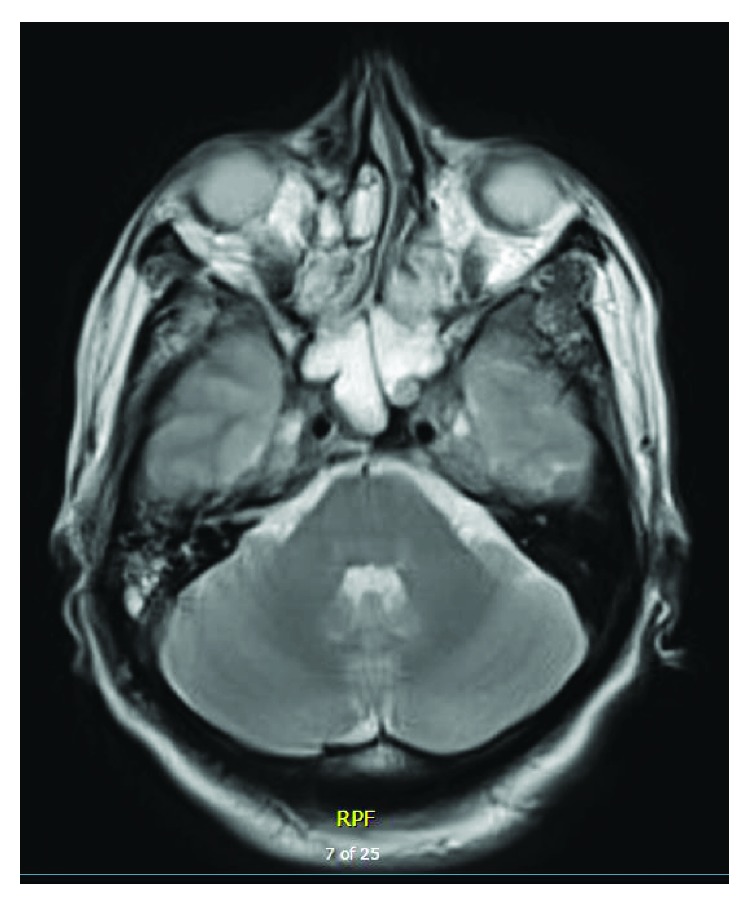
MRI showing sphenoidal and ethmoidal mucosal thickening.
